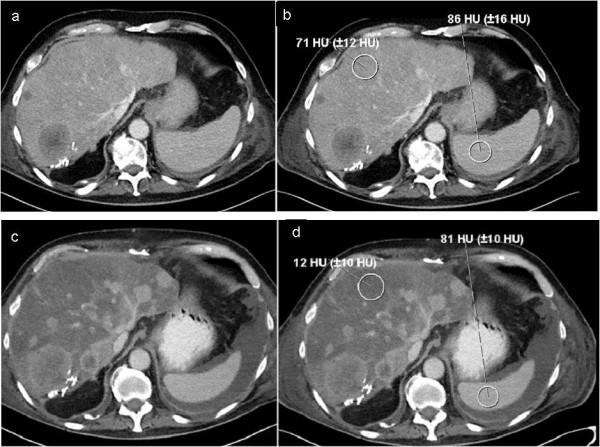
Pre-therapy and follow-up computed tomography images. (a,b) The pre-therapy axial post-contrast computed tomography images (with region of interest measurements) shows multiple liver metastases that were hypodense compared with the normal density of the liver and spleen parenchyma. (c,d) In the follow-up examination, a progress of the metastases was noted and a diffuse abnormal low density of the liver. HU, Hounsfield units.
